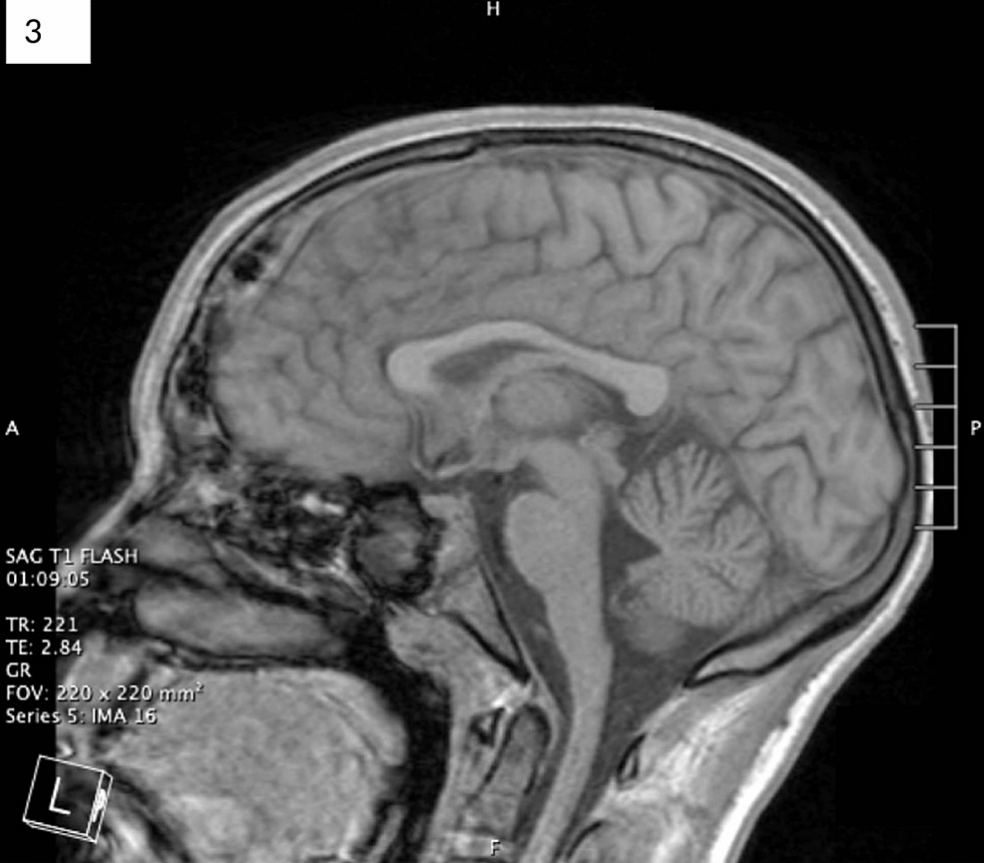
Final brain magnetic resonance imaging on hospital day 21 before the patient was discharged home. Imaging revealed continued improvement in the areas of involvement from the midline over the high frontal convexity and multiloculated over the right frontal lobe.
